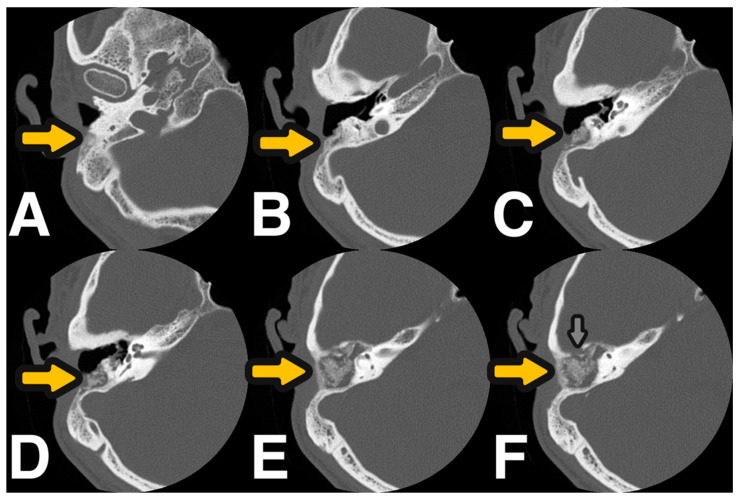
(A–F) Control HRCT-transverse plane scans of the right ear performed six months after mastoid obliteration surgery with use of sticky bone—yellow arrow. (F). Middle fossa bone destruction covered with sticky bone—grey arrow.
